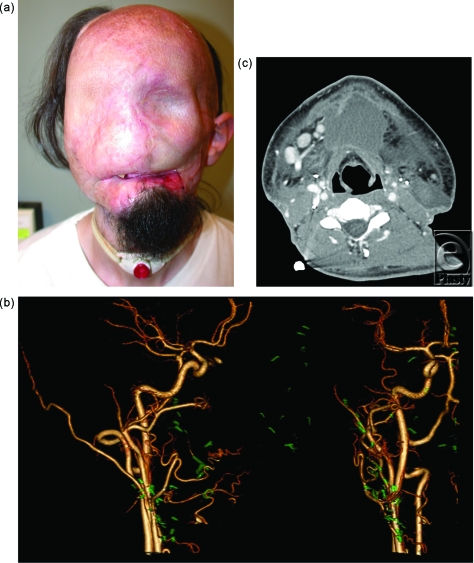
(*a*) Candidate 1 during initial evaluation for face transplantation. (*b*) Pure arterial images shown in 3-dimensional volumes after processing the 320 × 0.5 detector row computed tomographic (CT) images. The metal fragments, predominantly clips from the multiple surgical flaps, are separately segmented and denoted in green to show the proximity between the arteries and metal that must be identified for the surgical dissection. The figure demonstrates right cervical arterial system in 2 different viewing angles. (*c*) Axial slice from CT scan 13 days after face transplantation revealed abnormal fluid collection and subcutaneous edema in the submental region.
